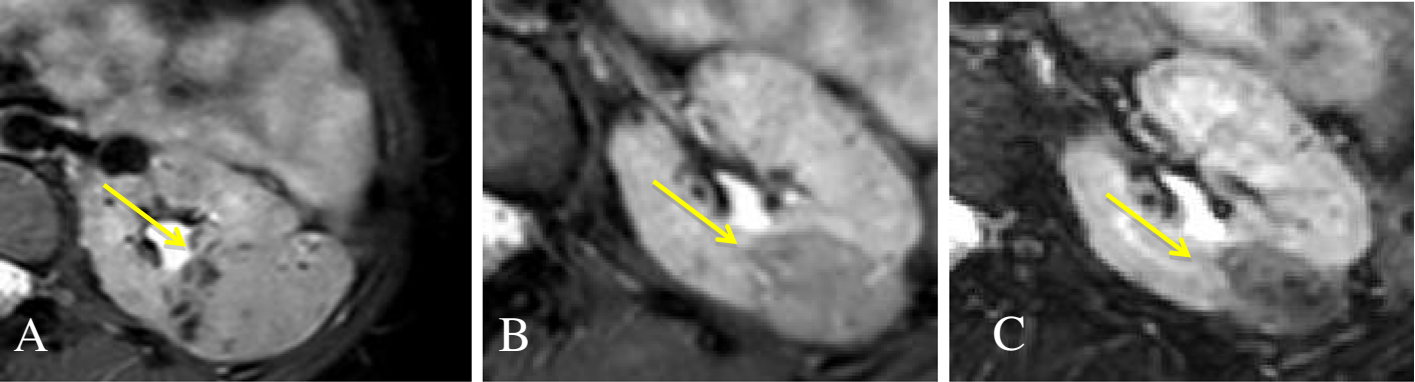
Progressive reduction of renal AMLs. A girl with a TSC2 mutation presented renal AMLs since childhood, with no impairment of renal function but with a progressivre growth both in number and in size. When she was 23 years old Everolimus was started (A, baseline), with a progressive reduction in the total volume of lesions. Figure B is the MRI after 12 weeks of treatment (76% of volume reduction), while figure C is after 144 weeks of treatment with Everolimus (85% of volume reduction).
